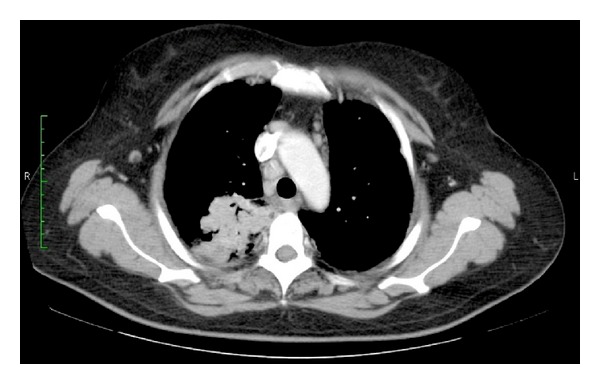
Computed tomography (CT) scan showing an irregular mass in the right lung field. This lesion was initially diagnosed as a cryptogenic organizing pneumonia.
